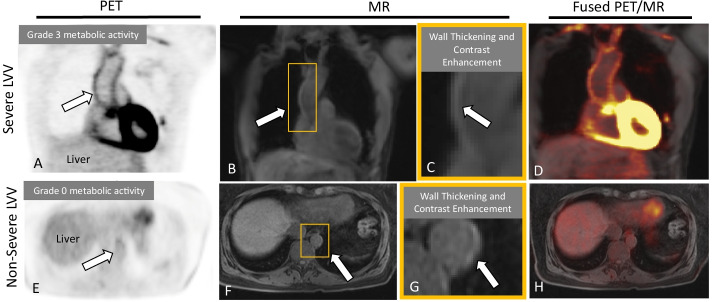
PET, MR, and PET/MR images. The MR, PET, and combined PET/MR findings in a patient with severe LVV (top row) presenting with a multi-year history of aching in bilateral legs and a stabbing pain in bilateral calves, and in a patient with non-severe LVV (bottom row), presenting with arthralgia and chronic constipation. Arrows point to an example vessel-of-interest in a severe LVV patient with positive PET and MR findings (at the ascending thoracic aorta) and in a non-severe LVV patient with positive MR findings (at the descending thoracic aorta). Positive MR findings more effectively ruled-in severe disease compared to PET, while negative PET findings more effectively ruled-out severe disease compared to MR
